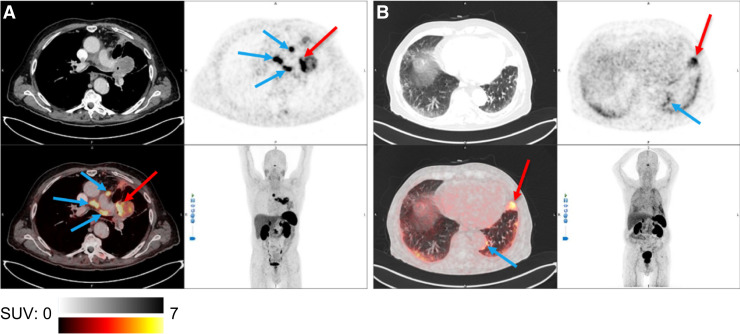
Examples of [^68^Ga]Ga-SSO120 PET/CT. Transversal CT (upper left), [^68^Ga]Ga-SSO120 PET (upper right), fused [^68^Ga]Ga-SSO120 PET/CT (lower left), and maximum-intensity-projection (lower right) images are shown. (A) High [^68^Ga]Ga-SSO120 uptake in primary SCLC tumor (red arrow; SUV_max_, 26.4) and mediastinal lymph nodes (blue arrows; SUV_max_, 19.4) 3 mo after end of 4 cycles of carboplatin plus etoposide. (B) High [^68^Ga]Ga-SSO120 uptake in primary LCNEC tumor (red arrow; SUV_max_, 8.1) and metastatic pleura (blue arrow; SUV_max_, 8.3) after third cycle of etoposide as monotherapy. (A and B) Physiologic uptake in pituitary gland, salivary glands, spleen, liver, adrenal glands, kidneys, and bladder is shown.
